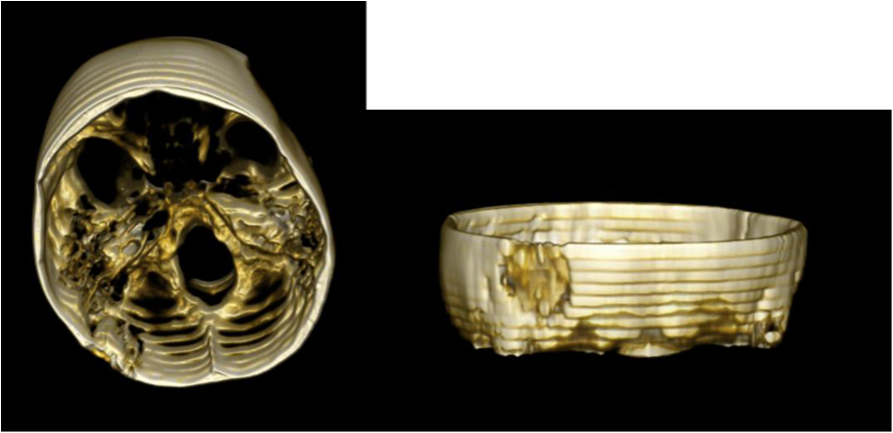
Three-dimensional computed tomography scan one day after first operation. Bone chips sealed in fibrin glue in the left retromastoid occipital bone.
